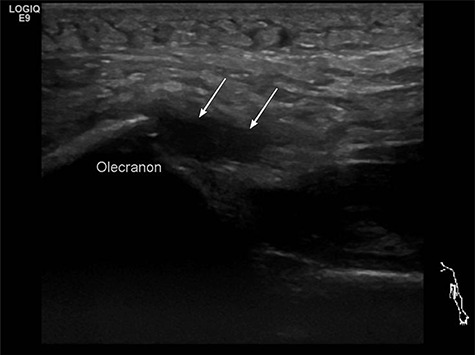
Preoperative ultrasound diagnostic.
